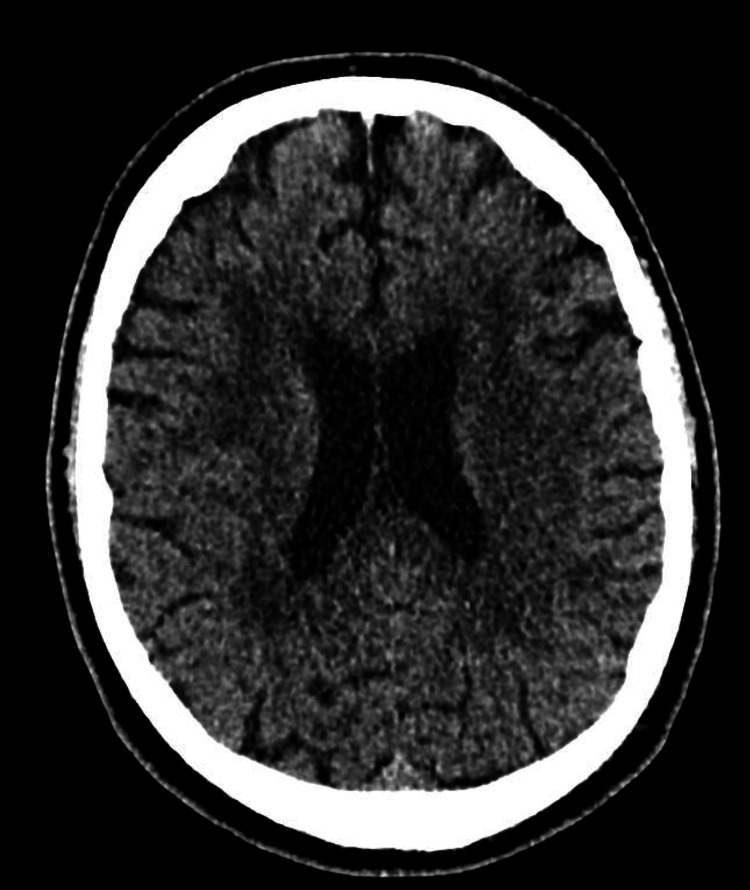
CT Head Non-contrast CT head at ventricular level demonstrating normal brain parenchyma without evidence of acute intracranial pathology, excluding central causes of neuromuscular weakness.
